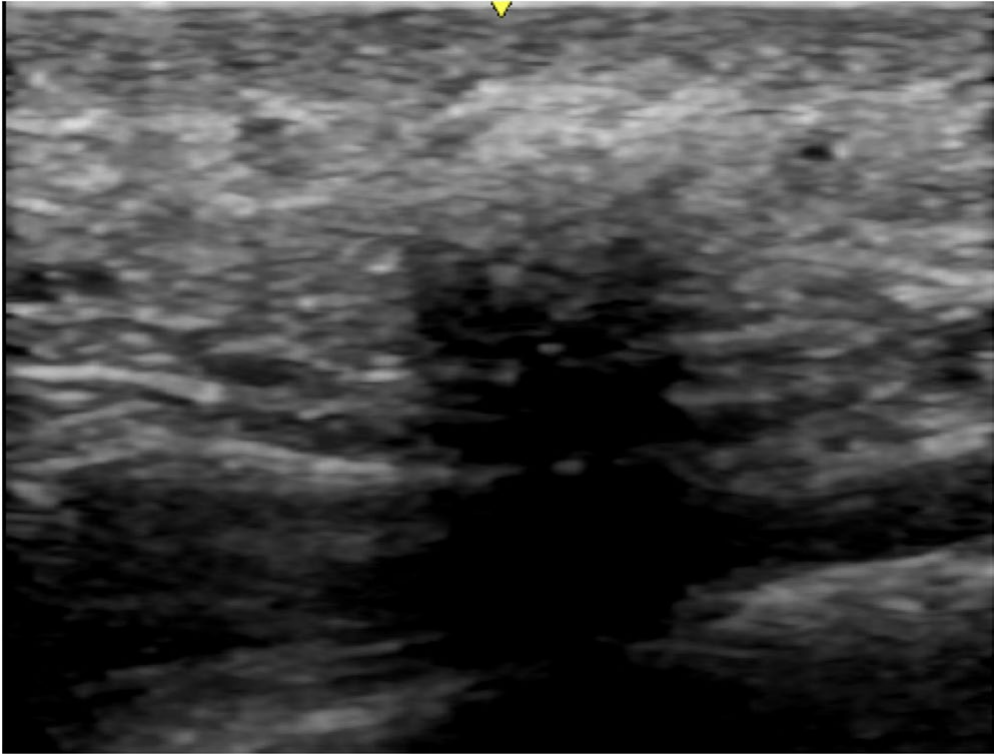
Ultrasound image of polymethylmethacrylate (PMMA) in the gluteal region. Subtle hyperechoic formation composed of multiple tiny hyperechoic deposits with a “comet‐tail” artifact, more clearly visualized using high‐frequency probes. This nodular formation is located very close to the superficial layers of the dermis and produces a posterior acoustic shadowing artifact. Note how this accumulation of PMMA is more discreet and presents a less hyperechoic surface compared to the nodule shown in Figure 8.
